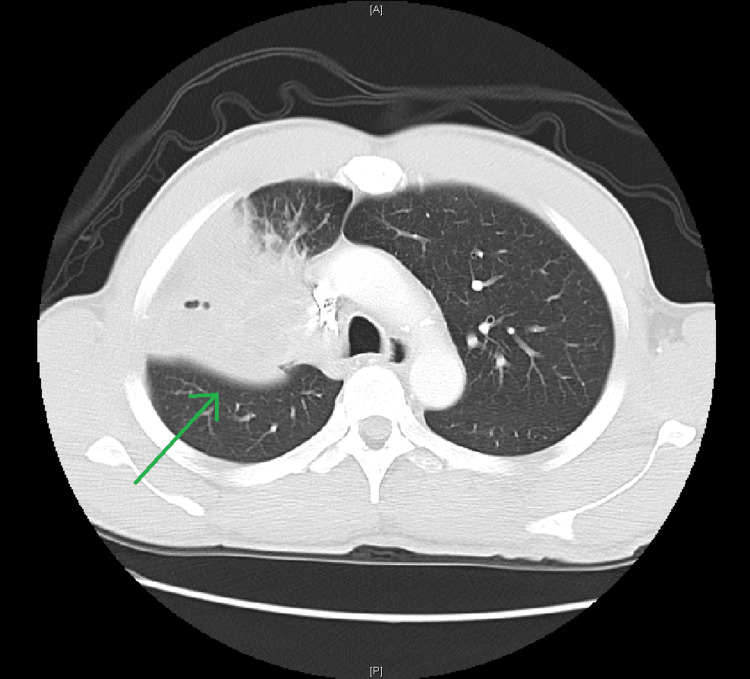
Computed tomography (CT) chest with intravenous contrast demonstrating the large suprahilar hypoattenuating lung mass (green arrow) causing right upper lobe bronchus occlusion and right upper lobe pulmonary artery narrowing
